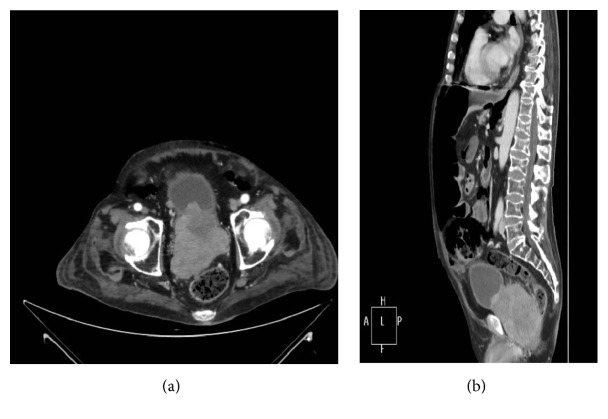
Computed tomography (CT) images, axial (a) and coronal (b), of the pelvis showing a huge mass involving bladder and rectum.
